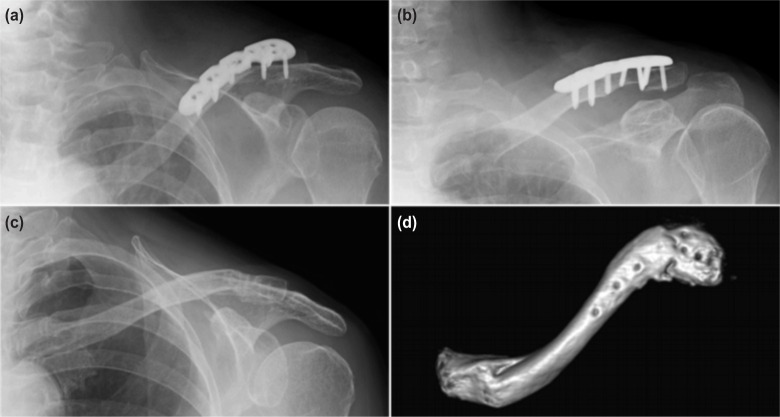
(a,b) Standard radiographs at four months after operation, (c) and after implant removal at 14 months after operation, (d) Three-dimensional CT scan image after implant removal.
